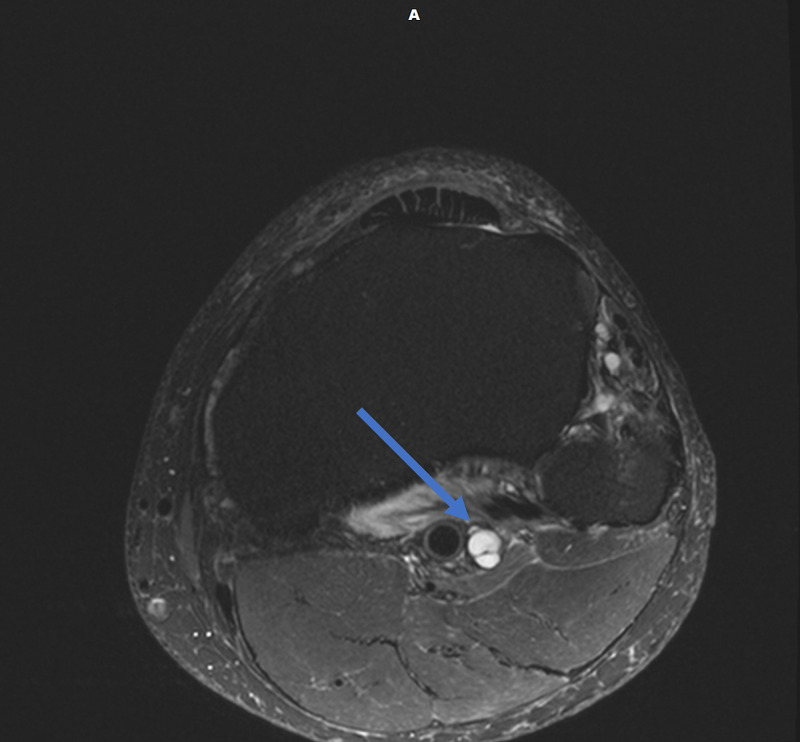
Axial T2-weighted TSE fat-saturated image demonstrating cysts connecting to the posteromedial superior tibiofibular joint TSE, turbo spin echo
